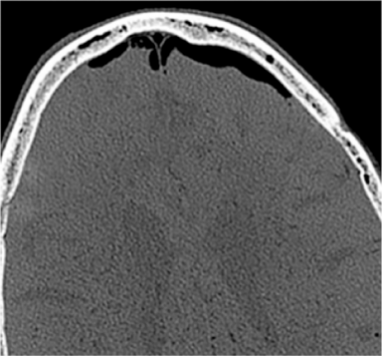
Pneu-encephalon with Mount Fuji sign in the axial CT scan
